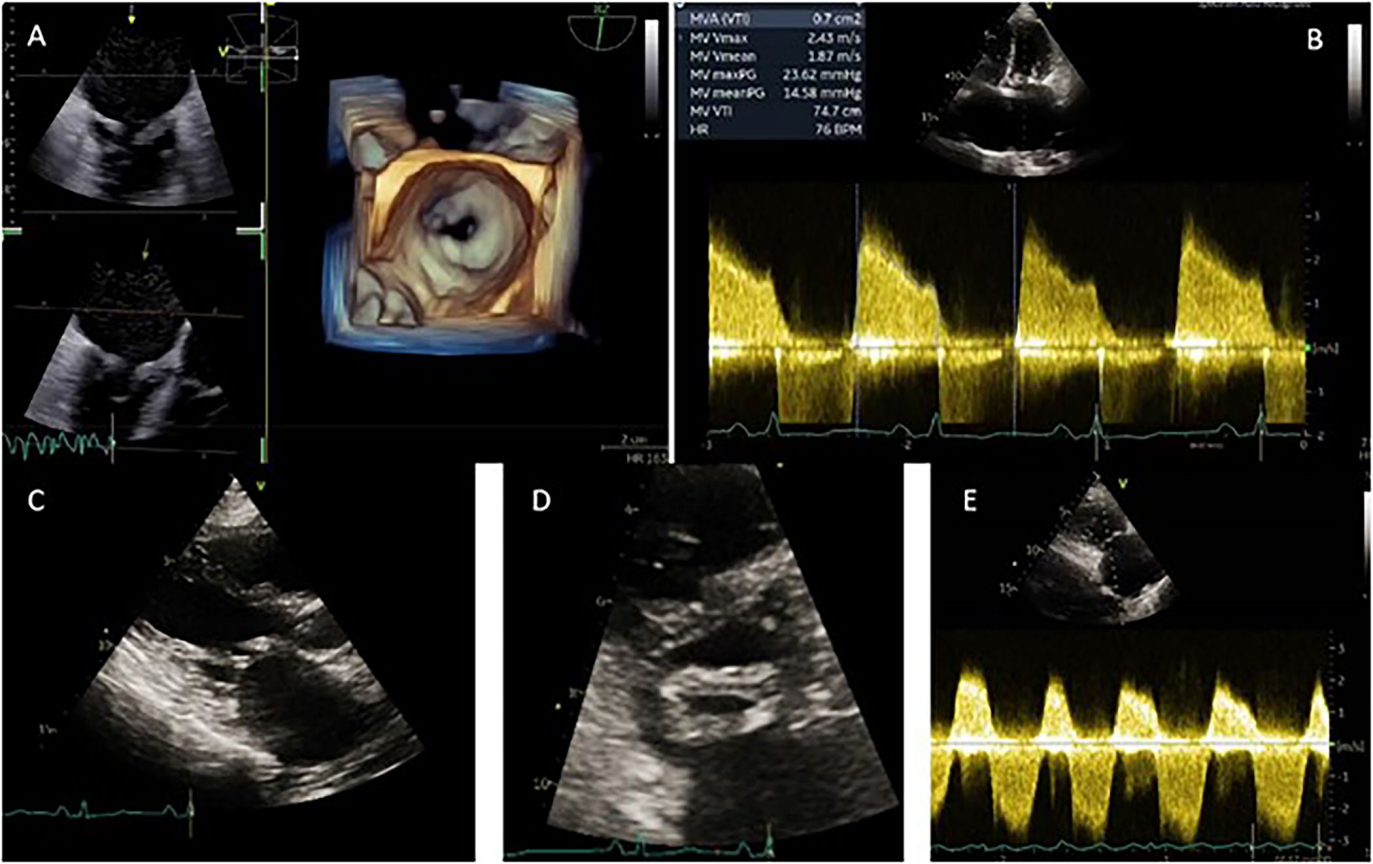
A pre‐procedure echocardiogram shows severe mitral valve stenosis with a mitral valve area of 0.8 cm² (3D‐image [A], 2D‐image [C, D]). Mitral valve mean gradient of 15 mmHg (B). Severe tricuspid regurgitation with right ventricular systolic pressure of 56 mmHg (E). [Color figure can be viewed at wileyonlinelibrary.com]
